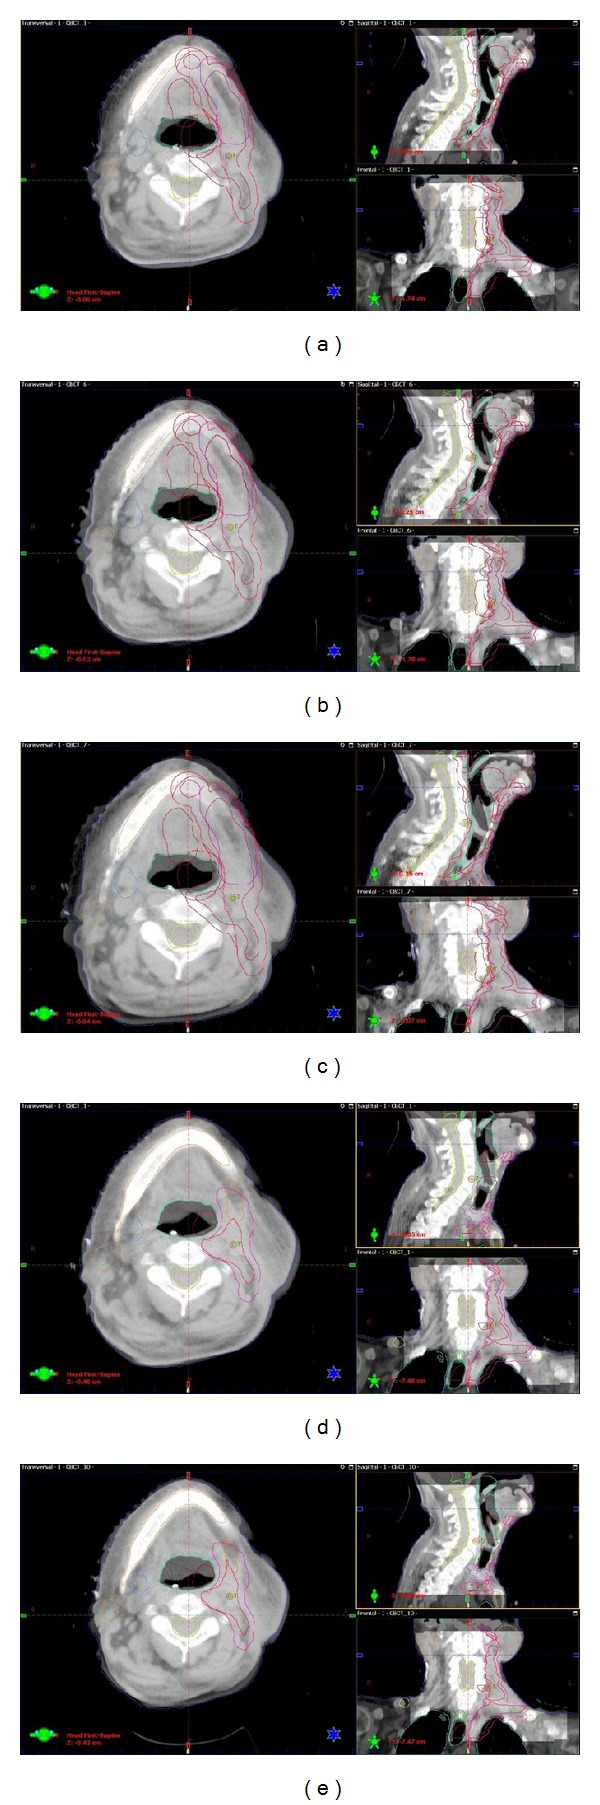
CBCT images of patient 1 on the first day of radiotherapy (a), first day of SRT medication (before medication) (b), 3 days later (c), 14 days later (adaptive radiotherapy) (d), and 28 days later (last day of radiotherapy) (e). Lymphedema improved 3 days later, but it got worse on one occasion. It improved 28 days later.
